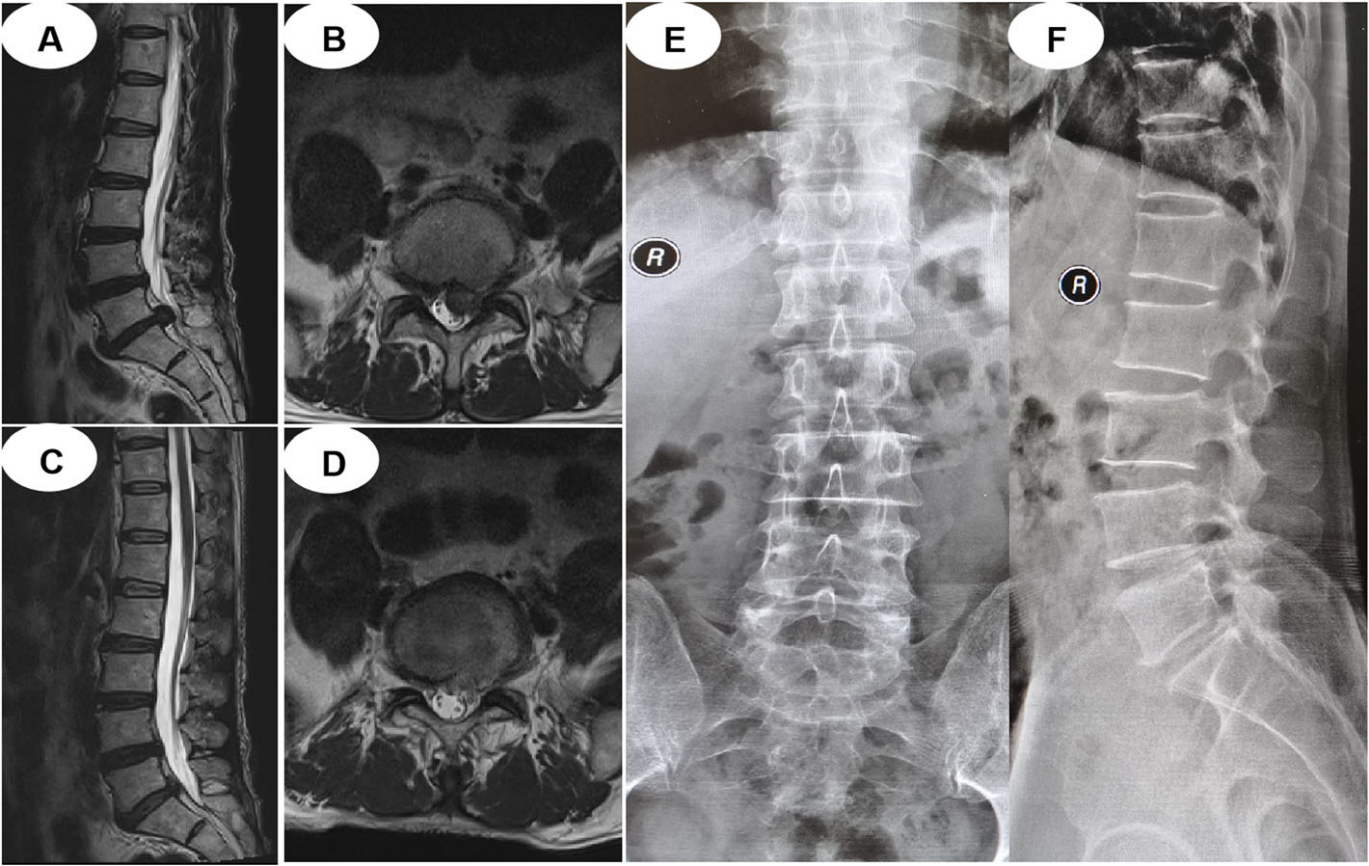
a and b MRI show L5-S1 discal hernia before MED; c and d MRI show the herniated nucleus pulposus is removed after surgery; e and f Postoperative x-ray images
